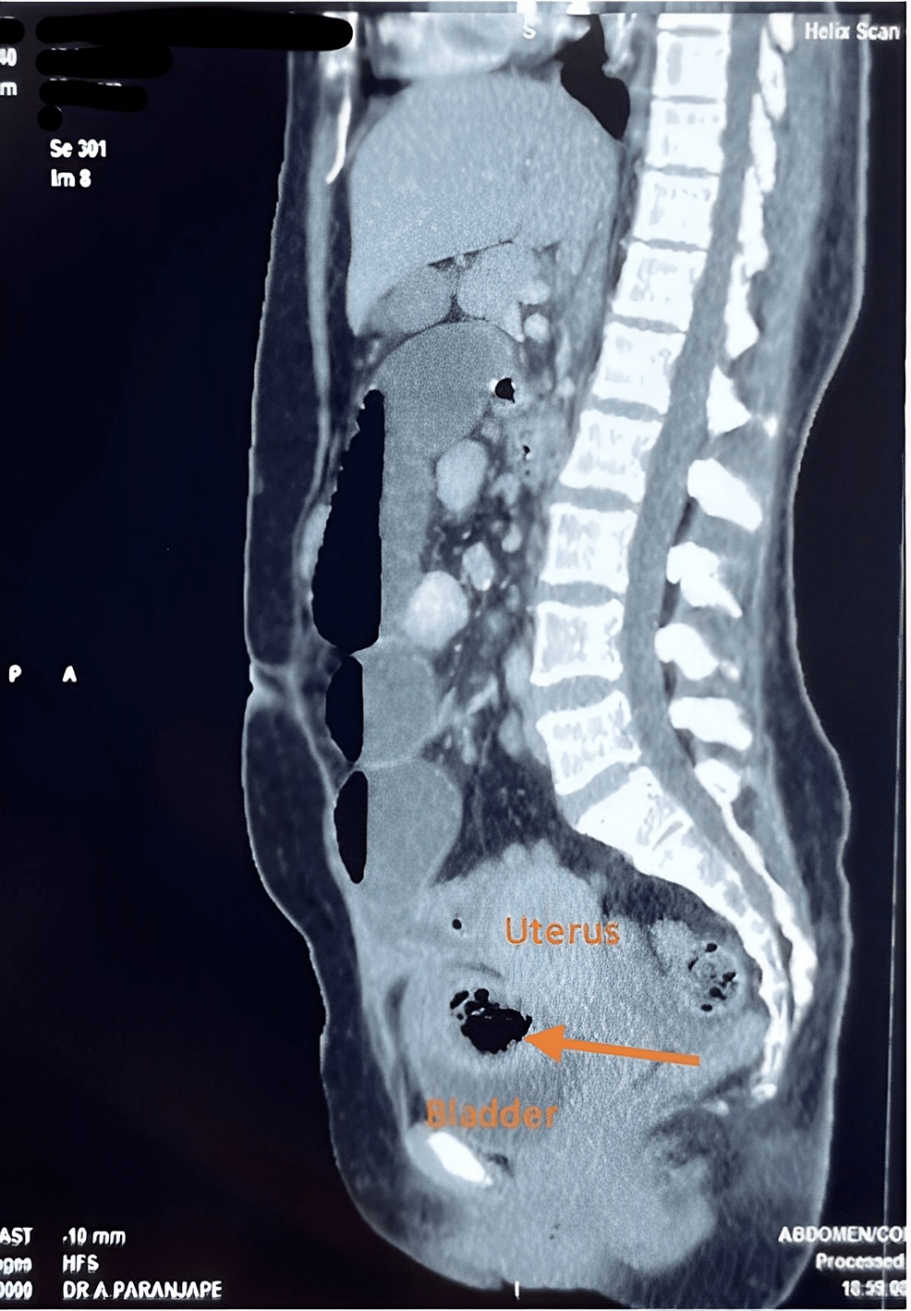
CECT of the abdomen and pelvis showing an oblong low-density structure (orange arrow) encapsulated between the bladder and the uterus, adhering to the bowel. CECT, contrast-enhanced computed tomography
